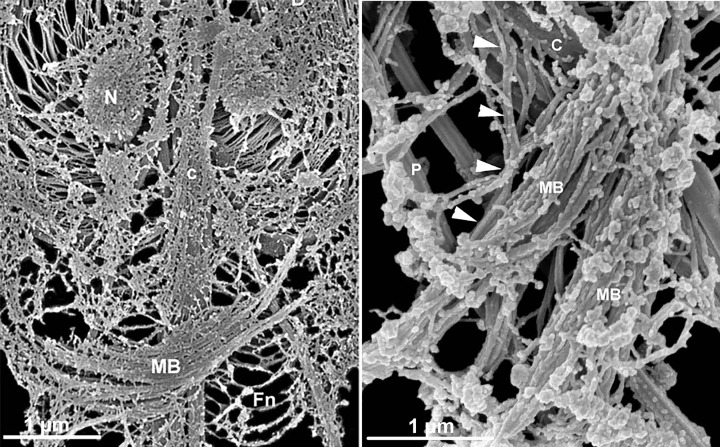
High-resolution SEM images of the median body cytoskeleton (A) The median body (MB) appears curved and oriented toward the cell's anterior region. (B) Individual microtubule fascicles that constitute the median body (MB) are clearly observed. N - Nucleus; C - Caudal flagella; P - Posterior-lateral flagella; Arrowheads - Funis microtubules. Images from the authors [147] with permission from Wiley Copyright Transfer Agreement (CTA).
